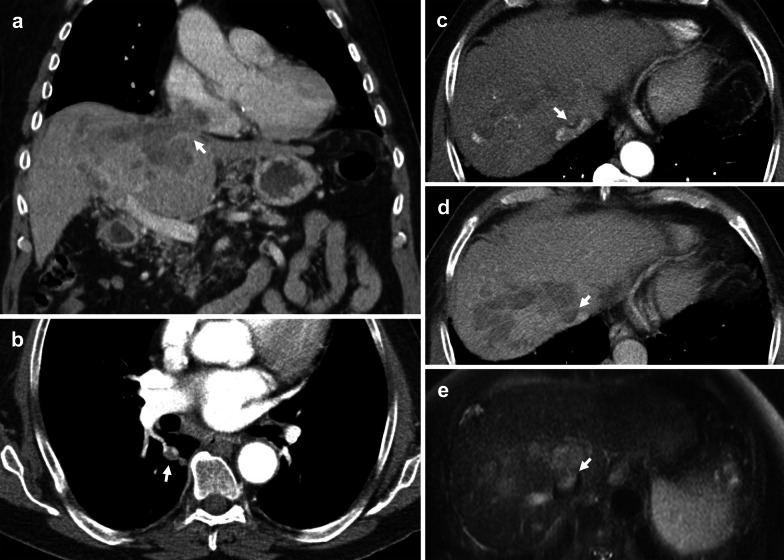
Hepatocellular carcinoma with IVC tumour thrombus, direct invasion of the right atrium and embolic tumour thrombus. a Contrast-enhanced coronal CT depicts direct invasion of the HCC (arrow) into the right atrium. b Axial CT chest shows subsegmental right pulmonary artery dilatation with internal filling defect (arrow), likely due to embolic tumour thrombus. c Axial CT shows multifocal arterial enhancement in the liver, predominantly in segment 7 and 8. The IVC tumour thrombus also demonstrates arterial enhancement (arrow), d is visualised as a partial filling defect (arrow) in portal venous phase, and e shows intermediate T2 signal (arrow) with extension into the hepatic IVC via the middle hepatic vein
